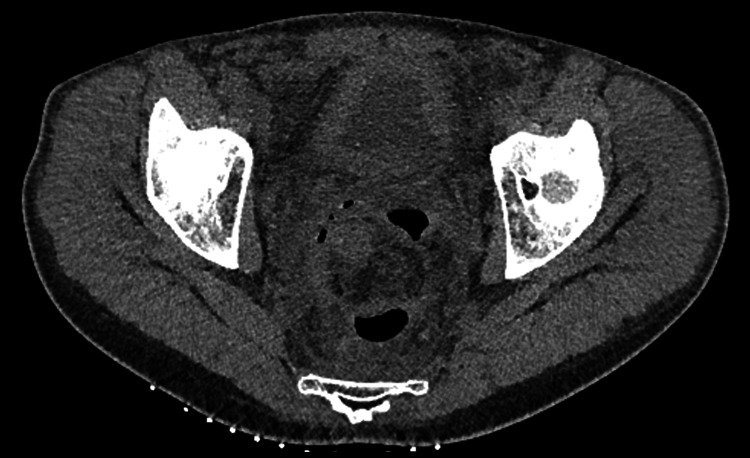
A planning pre-procedural CT of the abdomen and pelvis, just prior to drain insertion 17 days after the Altemeier procedure. Gas in the collection resembles an anastomotic leak (Axial).
